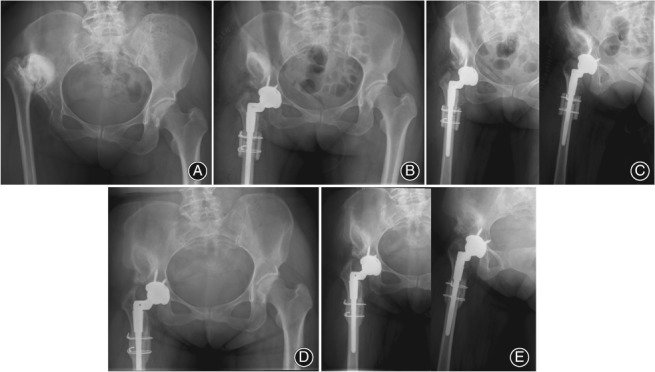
A 42‐year‐old woman with Crowe type IV high hip dislocation secondary to pyogenic arthritis who had a quiescent period of 20 years between infection and right total hip arthroscopy (THA). (A) Preoperative anteroposterior (AP) pelvic radiograph. (B) Postoperative AP pelvic radiograph immediately after right THA. (C) Postoperative AP and oblique hip radiographs immediately after right THA. (D) Postoperative AP pelvic radiograph 7 years after right THA. (E) Postoperative AP and oblique hip radiographs 7 years after right THA.
